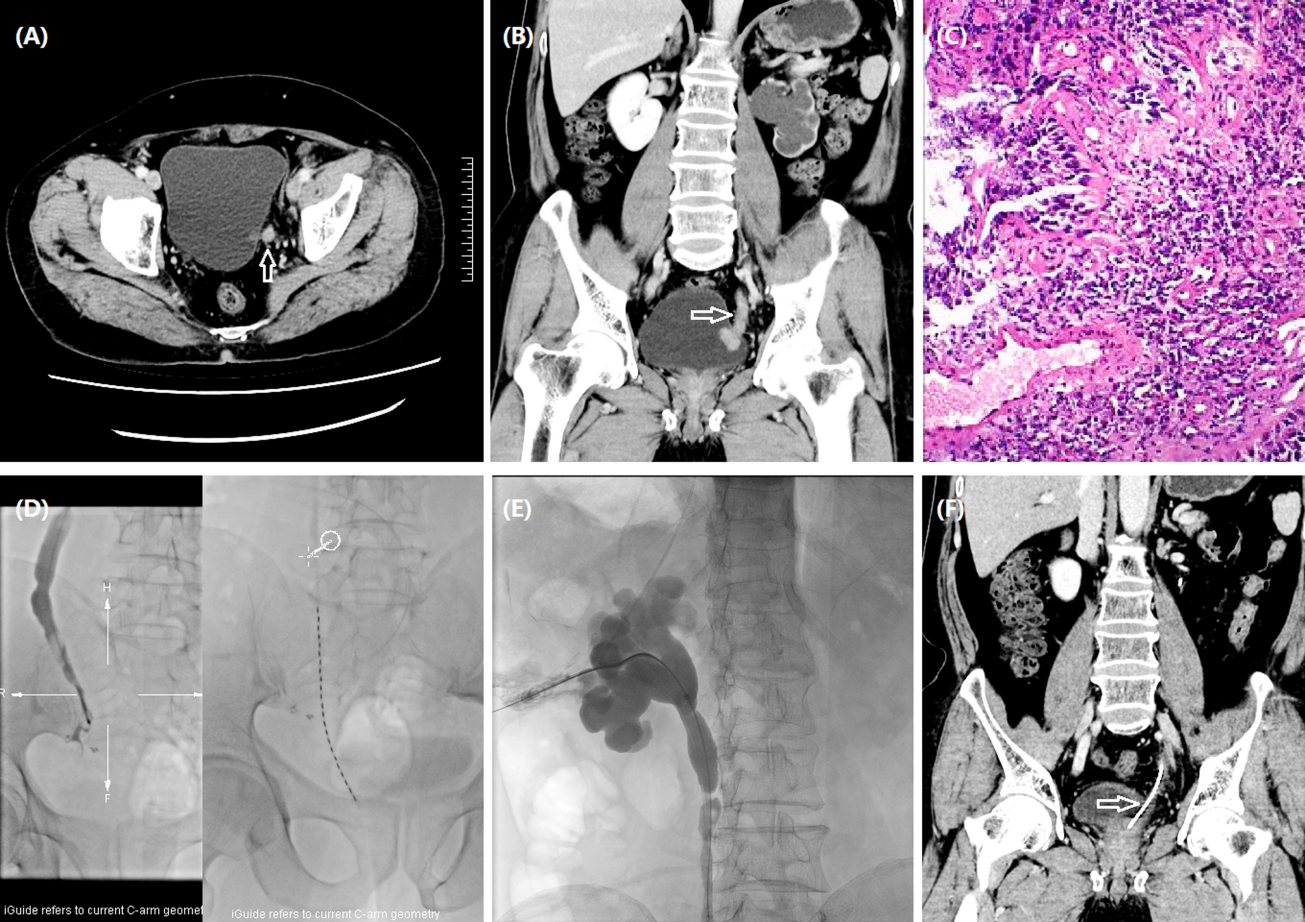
Iodine-125 seed strand placement for a 70-year man with ureteral carcinoma. (A) A left ureteral carcinoma (arrow) was shown by CT. (B) The tumor (arrow) was located in the left lower ureteric segment and bladder entrance with significantly dilated renal pelvis and thinning of renal cortex. (C) Pathology showed low-grade papillary urothelial carcinoma of the ureter without definite infiltration. (D) Urothrography revealed a 54.7-mm-long occlusion in the left lower ureter, and an iodine-125 seed strand with 23 seeds was inserted. (E) Nephrostomy was performed under fluoroscopic guidance. (F) Complete response (arrow) was detected by CT after 5.9 months.
